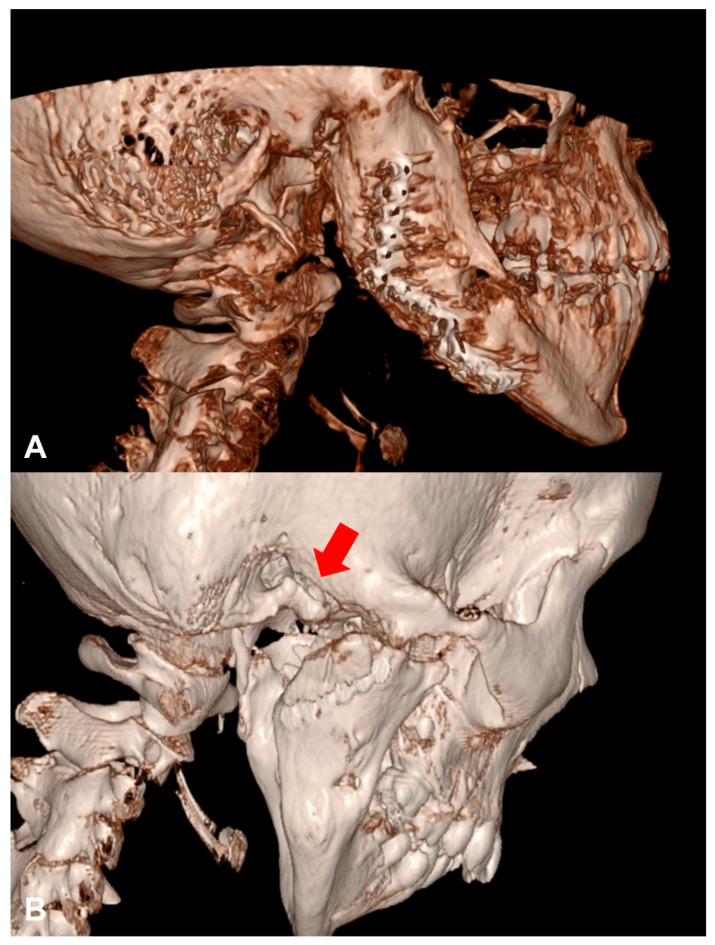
(A) Preoperative CBCT 3D reconstruction demonstrating full bony ankylosis of the right TMJ. (B) Postoperative CT 3D reconstruction demonstrating right bony external acoustic meatus perforation (red arrow).
